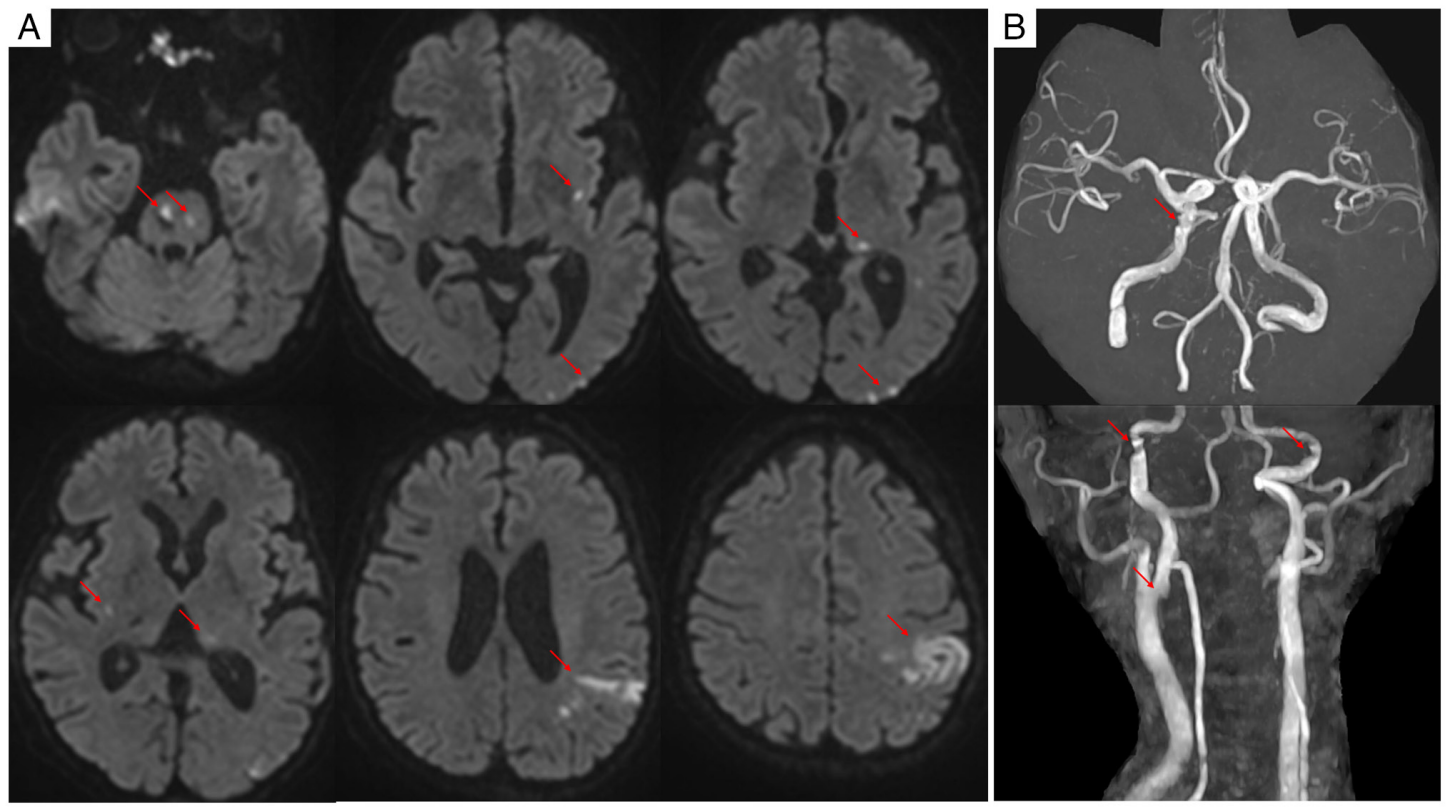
Neurological imaging findings. (A) Diffusion-weighted magnetic resonance imaging revealing multiple acute infarctions involving the pons, bilateral basal ganglia, left thalamus and left parieto-occipital region (arrow). (B) Magnetic resonance angiography showing vessel irregularities without obvious occlusion (arrow).
